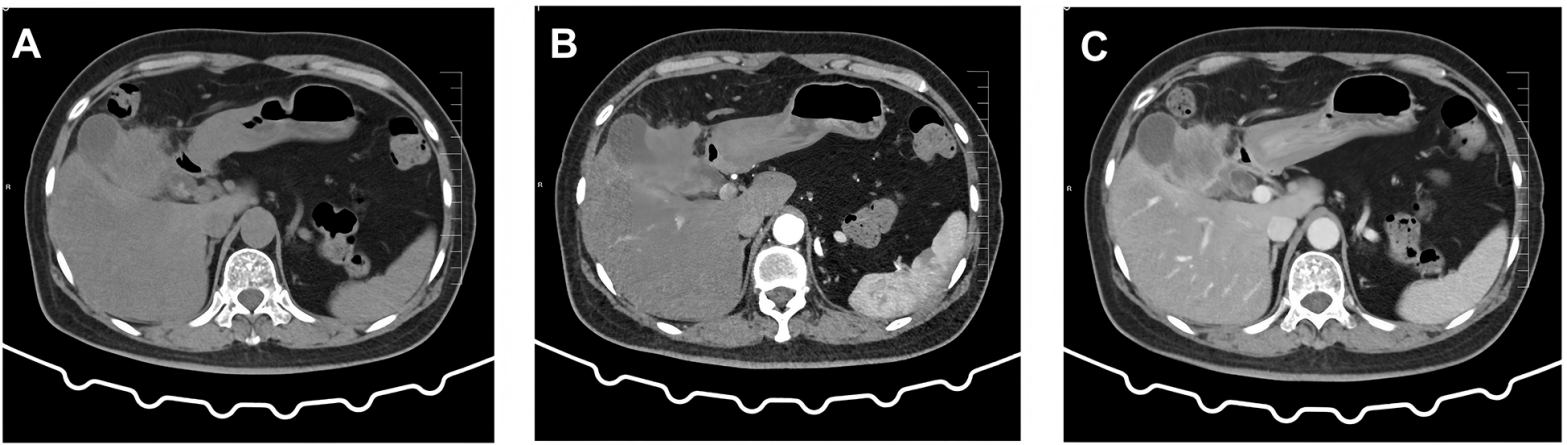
Preoperative abdominal CT findings. (A) Conventional scan reveals a low-density mass with indistinct borders in the gallbladder region. (B) Arterial phase scan shows heterogeneous enhancement in the surrounding area. (C) Venous phase scan demonstrates persistent but slightly diminished enhancement. The mass invades adjacent hepatic parenchyma (segments IVb and V), suggesting a localized gallbladder malignancy.
